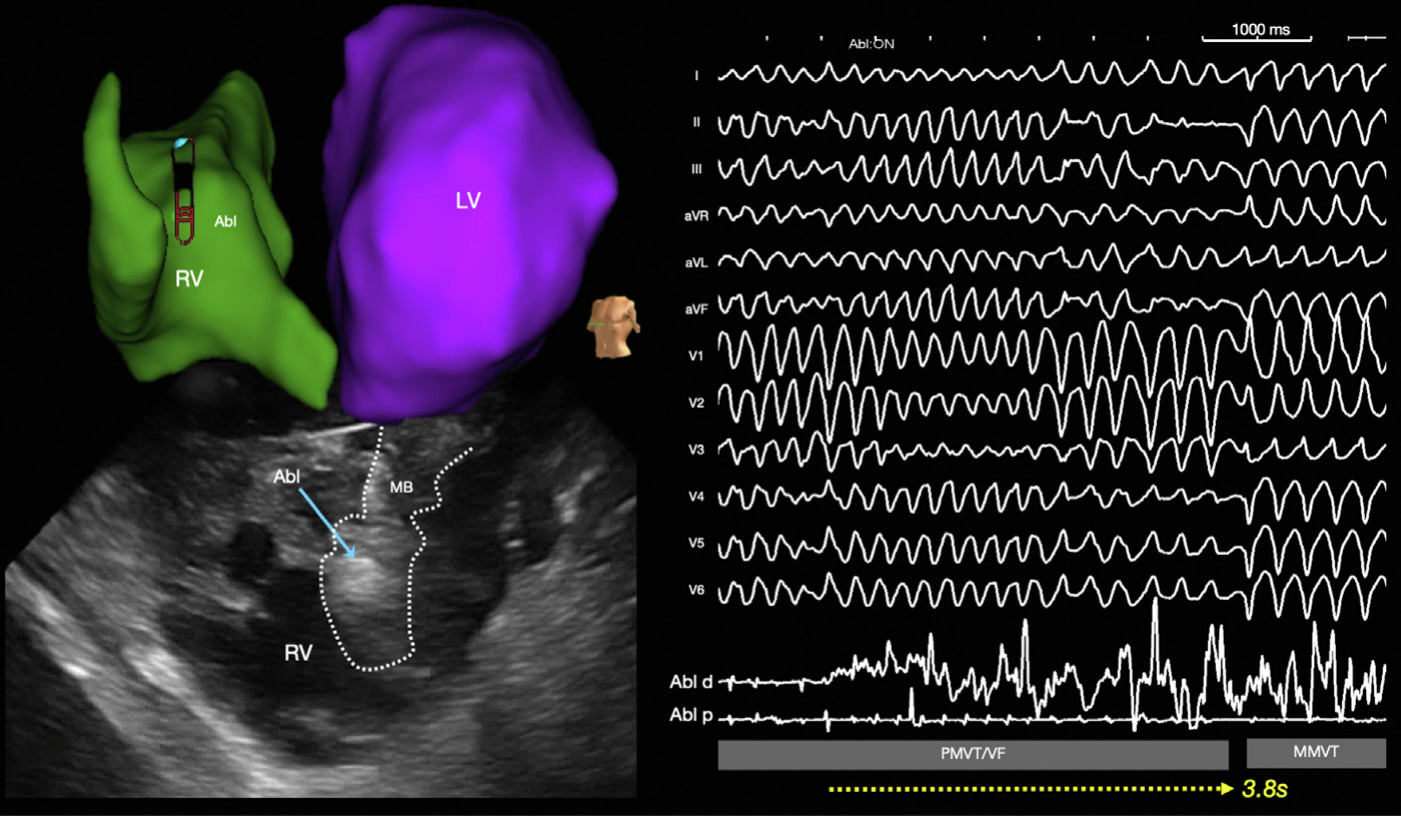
Anatomic Location of Ablation That Resulted in Termination of Ventricular Fibrillation From Moderator Band Electroanatomic map shows ablation catheter (Abl) within the right ventricle (RV). Intracardiac echocardiography confirmed contact with lesion formation (Abl) on the moderator band, which is an intracavitary structure within the RV. During radiofrequency energy delivery, sustained ventricular fibrillation (85 seconds) directly terminated to monomorphic ventricular tachycardia during the delivery of RF ablation in 3.8 seconds of ablation.
